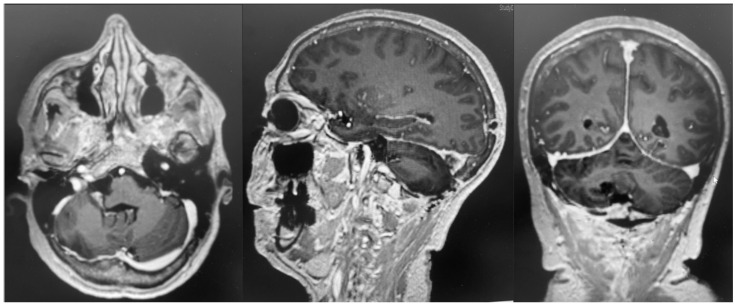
Postoperative MRI + c: these pictures show the gross total resection of the lesion with no signs of postoperative complications.
